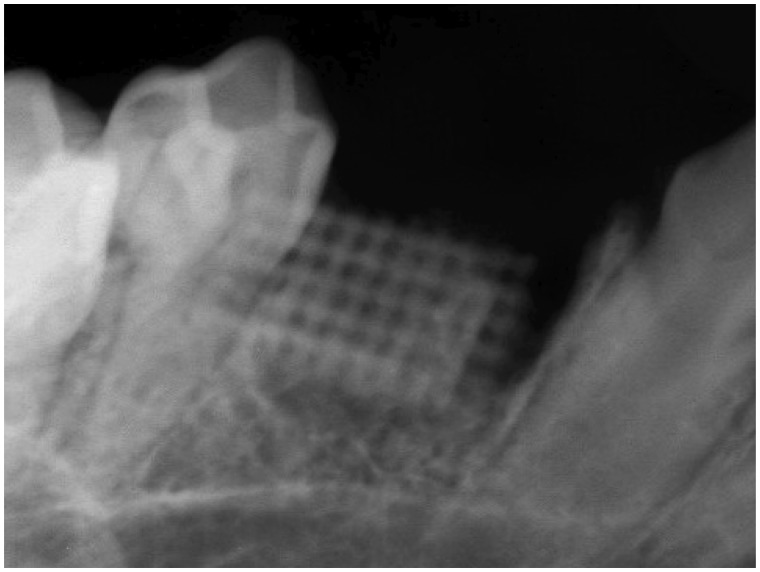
X-Ray examination of the scaffold immediately post-operation
